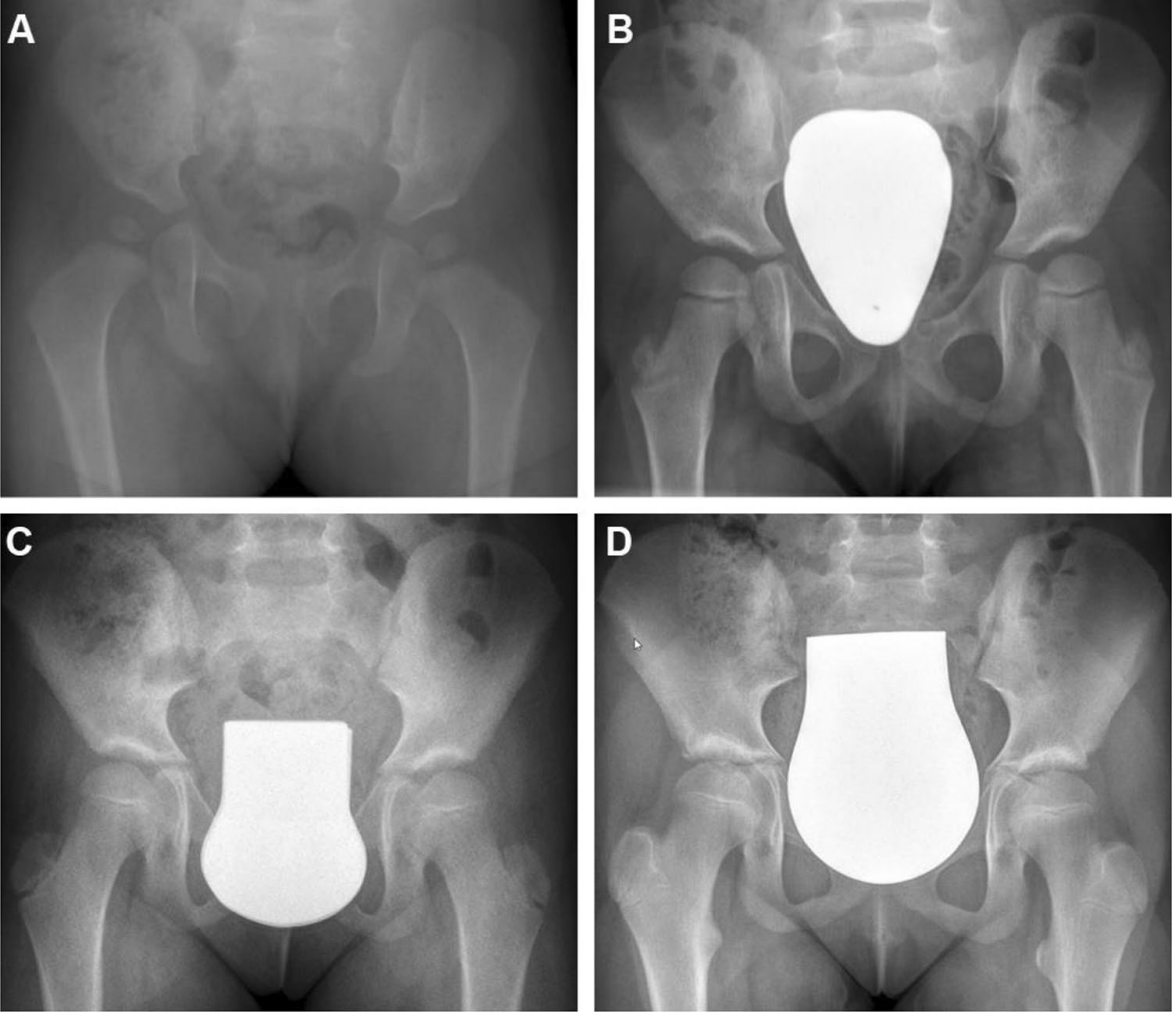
Radiological development of the patient of Fig. 3 with an initial hip type IV on the right side, A X-ray control at the age of 15 months: ACI: 26° (Tönnis classification: slightly dysplastic), B at the age of 48 months: ACI: 21° (Tönnis classification: normal finding), C at the age of 80 months: ACI: 18°, CEA 28° (Tönnis classification: normal finding), D at the age of 124 months: ACI: 11°, CEA: 30° (Tönnis classification: normal finding)
